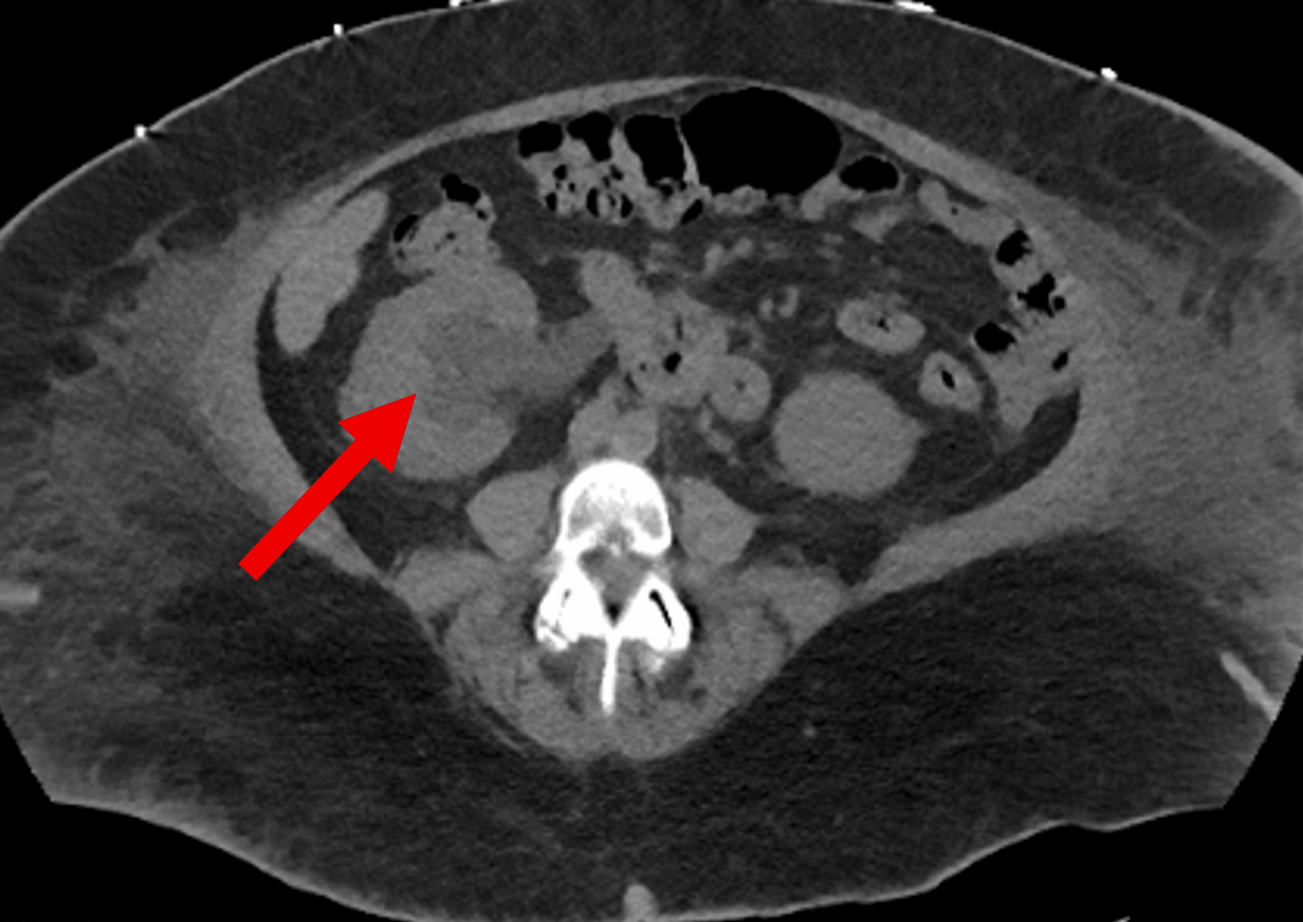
CT of the abdomen and pelvis (axial) without contrast demonstrating right-sided hydroureteronephrosis (red arrow). CT: computed tomography
